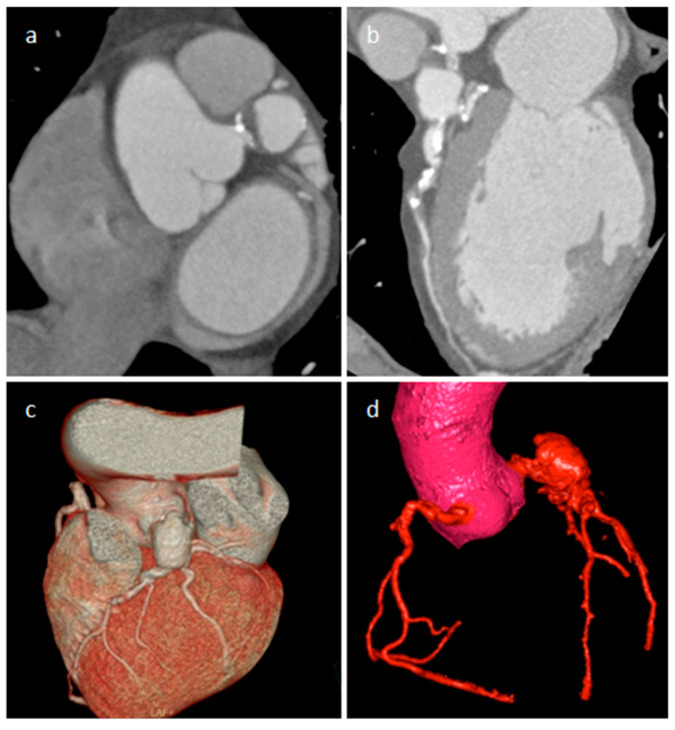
A 65-year-old male patient with a partially thrombosed giant aneurysm of the left main coronary artery on short-axis view (a), multiplanar reconstruction (b) and volume rendering (c,d) CT images.
